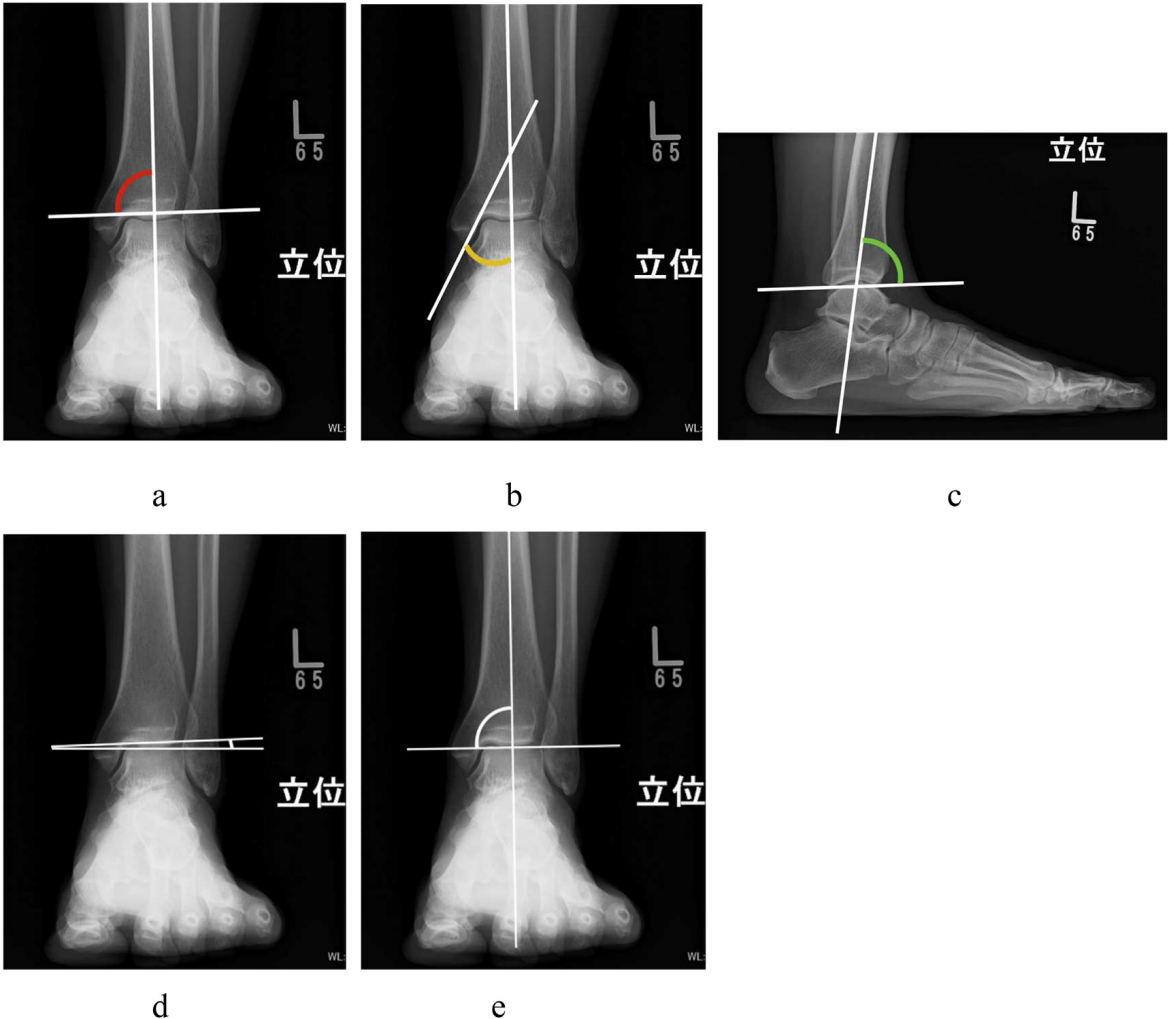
Radiological evaluation parameter on weight-bearing radiograph. a. Tibial articular surface angle (TAS): the angle between the axis of the distal one-third part of the tibia and the line along the articular surface of the tibial plafond (mean ± SD = 87.7 ± 3.0°^13^). b. Medial malleolar angle (MMA): the angle between the axis of the distal one-third part of the tibia and the line along the articular surface of the medial malleolus (mean ± SD = 23.0 ± 4.7°^13^). c. Tibial lateral surface angle (TLS): the angle between the axis of the distal one-third part of the tibia and the line joining the anterior and posterior edges of the tibial plafond(mean ± SD = 81.1 ± 2.2°^13^).d. Talar tilt angle (TTA): the angle between the line along the articular surface of the tibial plafond and the line along the superior surface of the trochlea of the talus (normally less than 4°^16^). e. Tibiotalar surface angle (TTS): the angle between the axis of the distal one-third part of the tibia and the line along the superior surface of the trochlea of the talus (normally about 90°).
